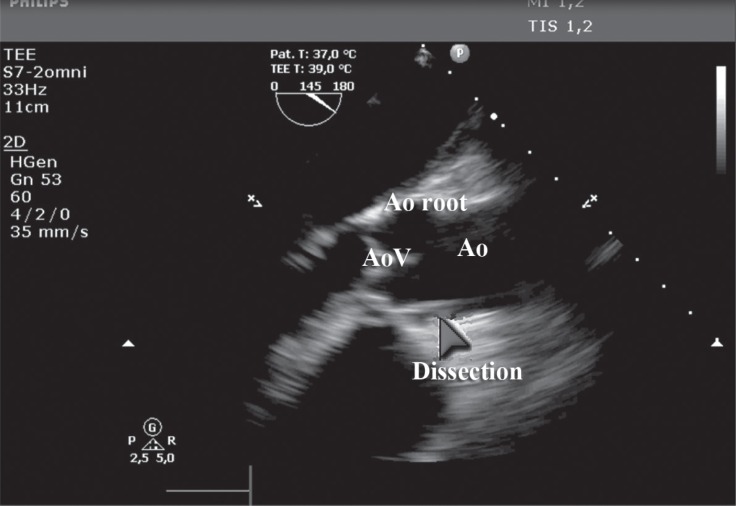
Transesophageal echocardiogram (long axis) – arrow showing the aortocoronary dissection
